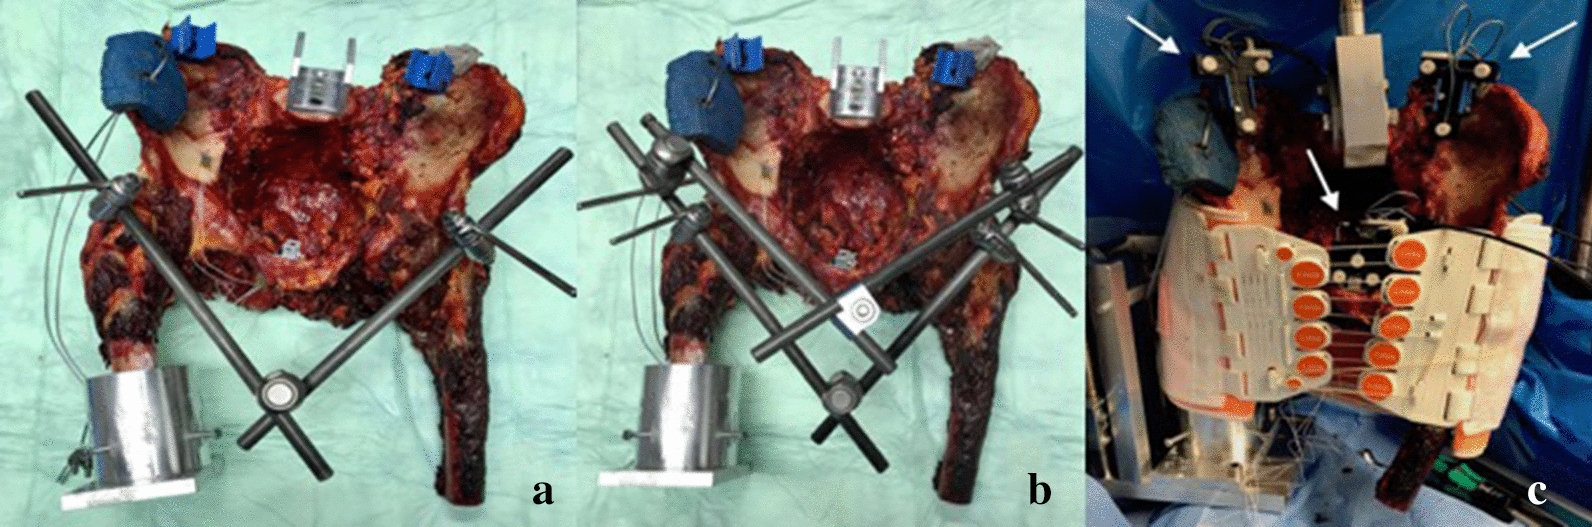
a–c: Experimental setup. Supraacetabular external fixator with one pin (a) and two pins (b) on each side. T-POD applied on the cadaveric pelvis (c) with arrows indicating the sensors of the 3D-ultrasound measuring system
